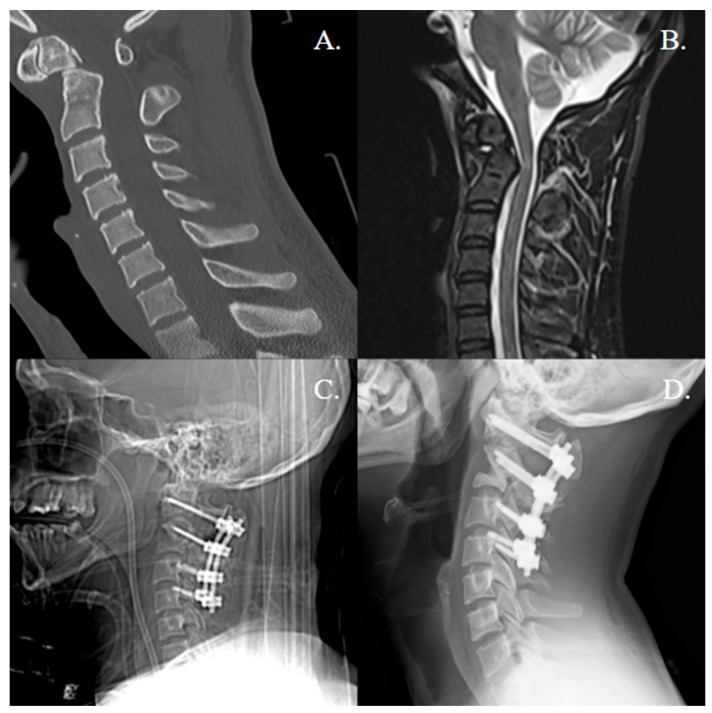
Odontoid fracture (motor-vehicle collision): pre-op imaging, construct, and fusion. (A) Sagittal cervical CT showing a Type II odontoid fracture at the base of the dens. (B) Pre-operative sagittal T2-weighted MRI demonstrating posterior protrusion of the fractured odontoid into the spinal canal without cord signal change. (C) Immediate post-operative lateral radiograph showing C1 lateral mass and C2 pedicle/translaminar screws connected with rods, with appropriate reduction and hardware position. (D) One-year post-operative lateral radiograph demonstrating maintained alignment, and no hardware lucency, consistent with stable arthrodesis.
